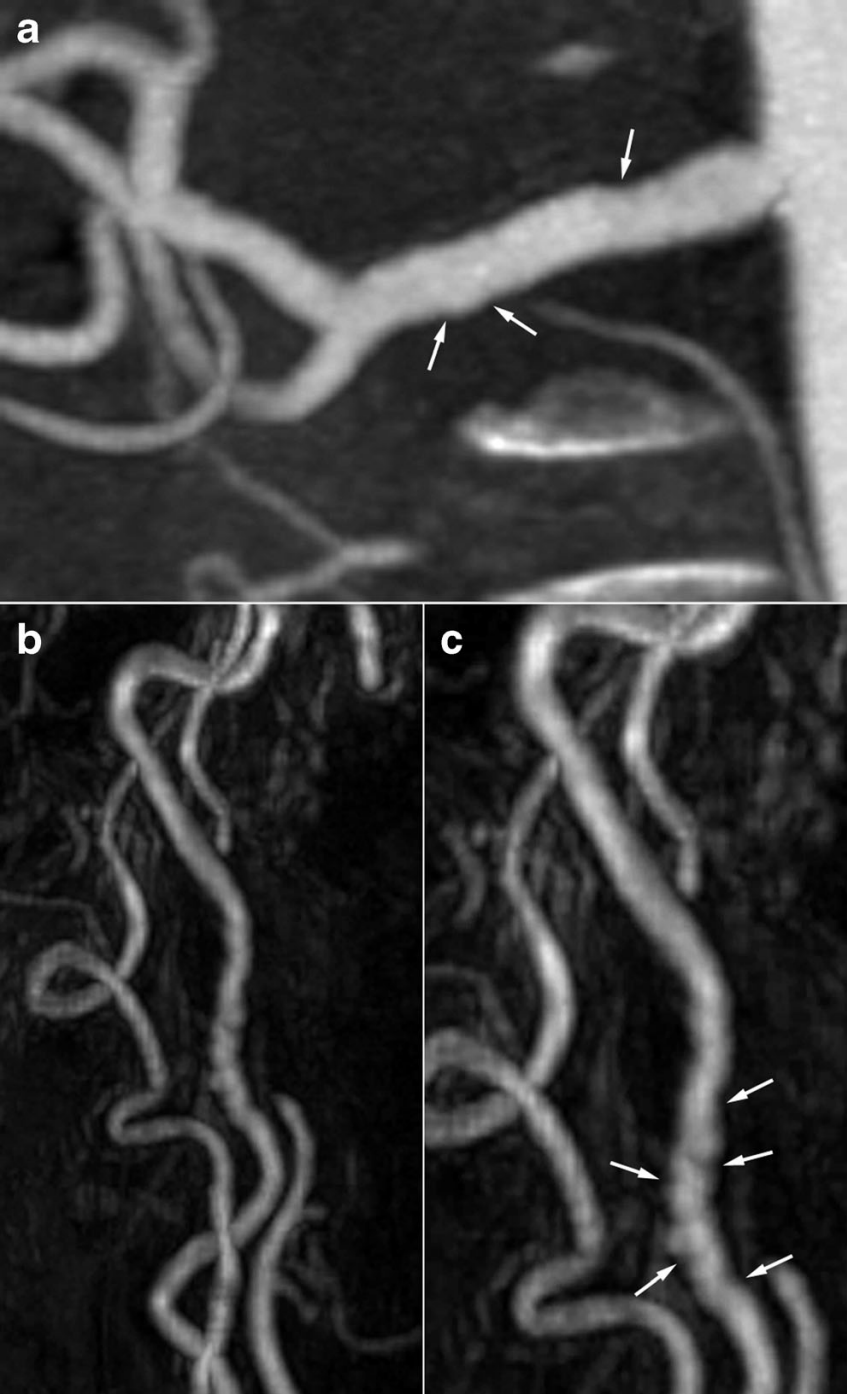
Radiologic findings in a 68-year-old female patient who suffered from an inferior myocardial infarct secondary to spontaneous dissection of the right coronary artery. a Renal coronal CTA MIP reconstruction showing moderate involvement of the right renal artery (*white arrows*). b and c Three-dimensional gadolinium-enhanced MRA reconstructions in the same patient showing the typical string-of-beads aspect (*white arrows*) of the internal carotid artery, highly suggestive of FMD
